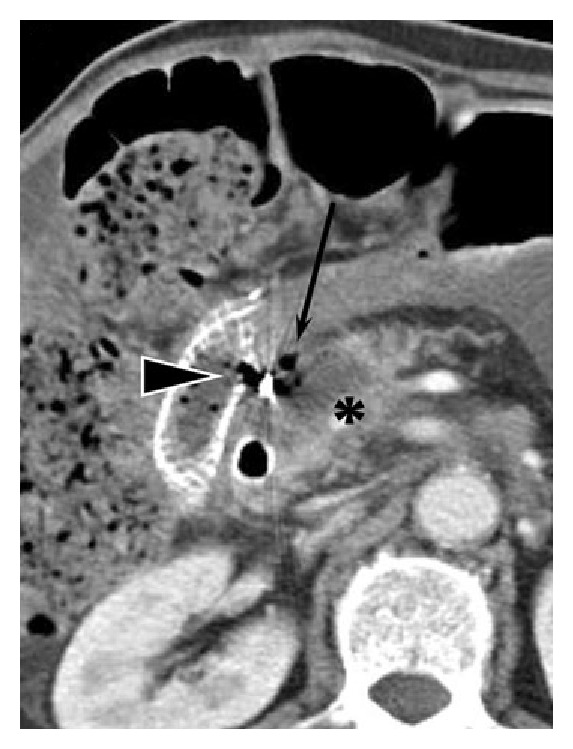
Necrosis (asterisk) in the pancreas head with rim enhancement, a fistula between the pancreatic tumor and the adjacent bowel with the mottled air densities (long thin arrows), and communication between the duodenum and the ablated cavity via focal disruption of the duodenal stent (arrowhead) in a follow-up CT after HIFU ablation (used with permission [7]).
